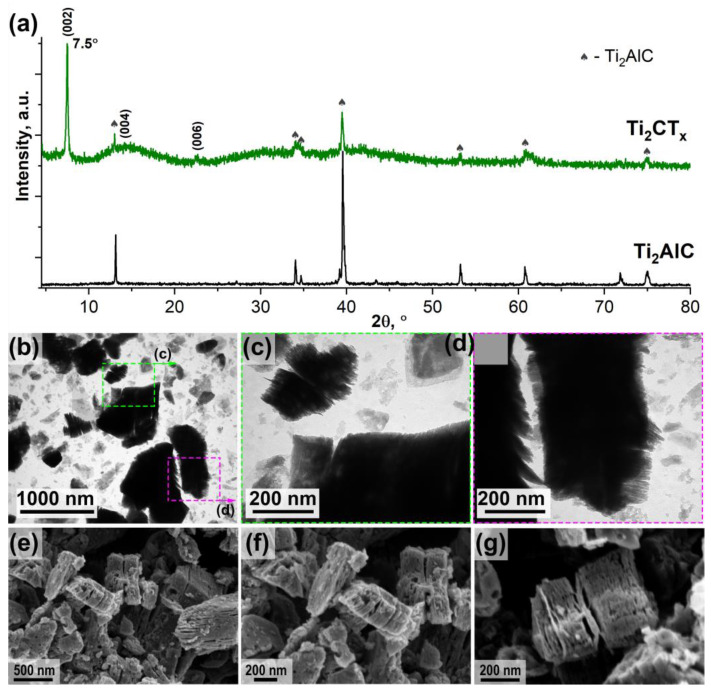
The X-ray powder patterns of the original Ti_2_AlC MAX-phase and the obtained Ti_2_CT_x_ MXene (a) and the Ti_2_CT_x_ MXene microstructure particles according to TEM (b–d) and SEM (e–g).
